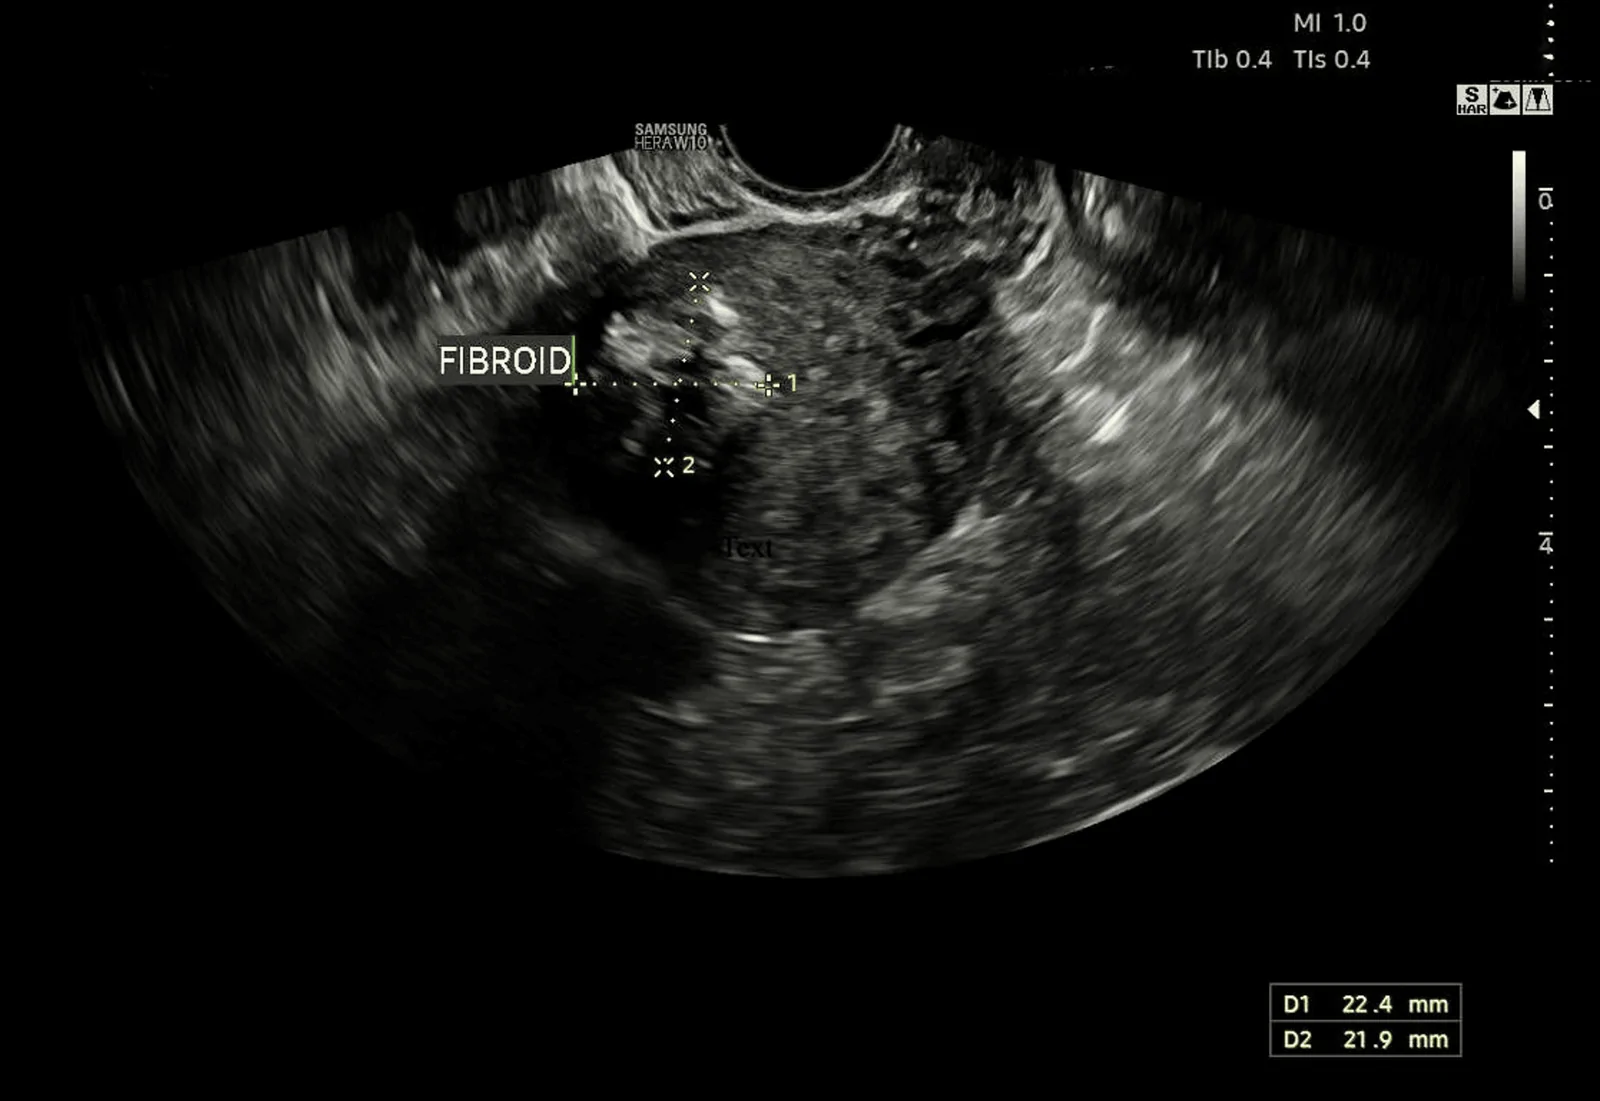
Final follow-up ultrasound showing a small calcified intramural fibroid. Subsequent follow-up transvaginal ultrasound performed three months after the previous scan, demonstrating a small calcified intramural fibroid measuring approximately 22 × 21 mm adjacent to the endometrial cavity.
